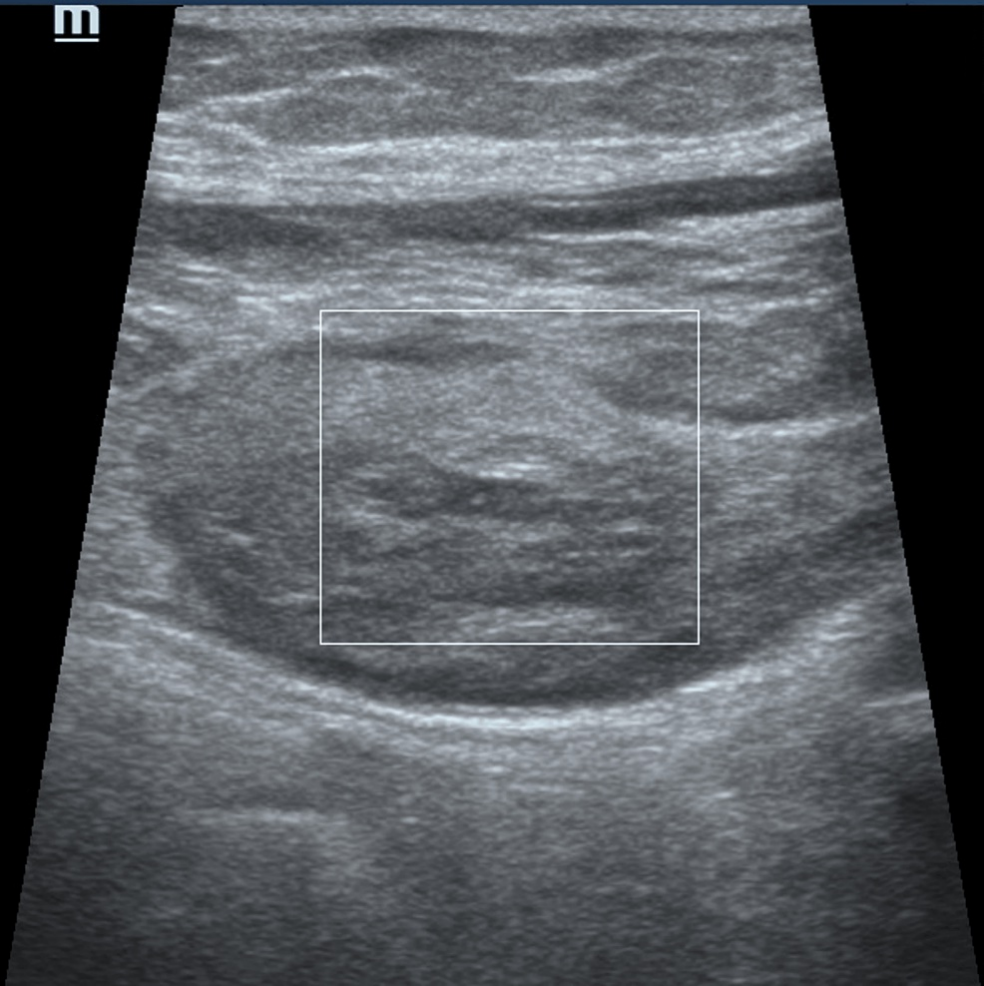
Doppler USG image showing no vascularity region of interest in the lesion (Patient 10)
